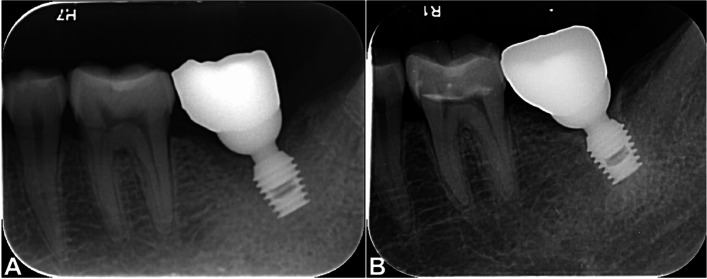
Peri-implant bone appears markedly denser around the implant after 3 years of loading. A. Initial radiograph. B. Radiograph after 3 years of loading
